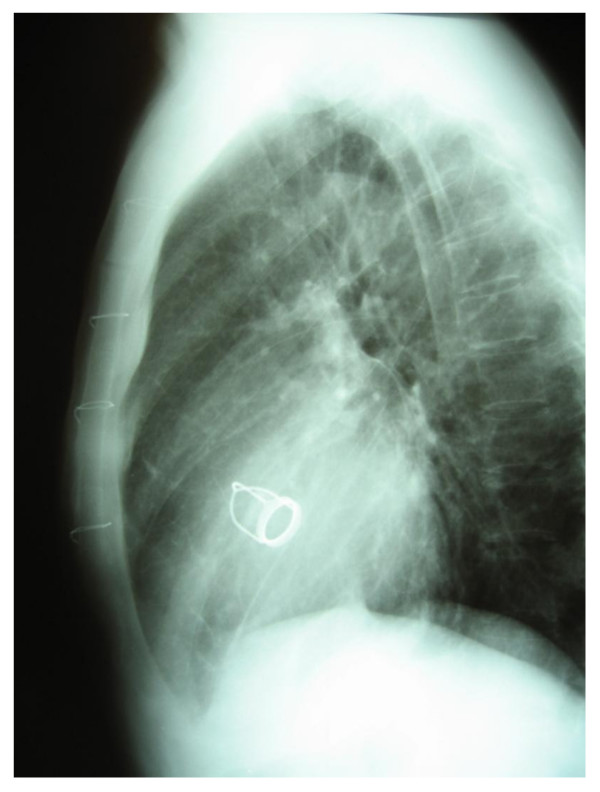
Pre-operative chest X-ray with the Starr-Edwards-caged-ball-valve in aortic position.
